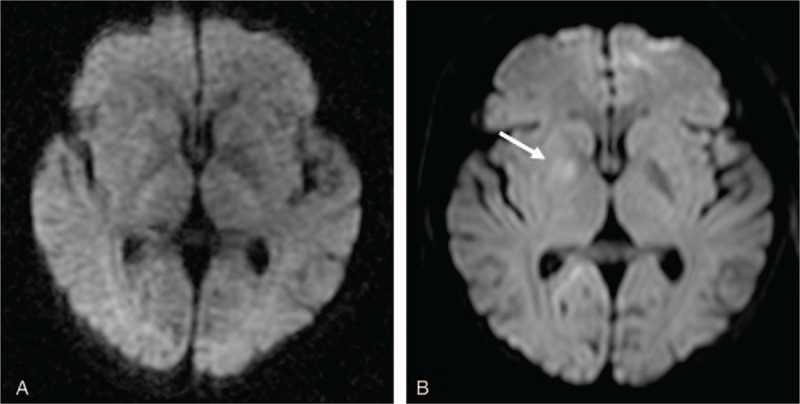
A, Initial diffusion-weighted MRI at presentation shows the absence of restricted diffusion. B, Diffusion-weighted MRI after deterioration of the symptom shows restricted diffusion in the right globus pallidus (arrow). MRI = magnetic resonance imaging.
